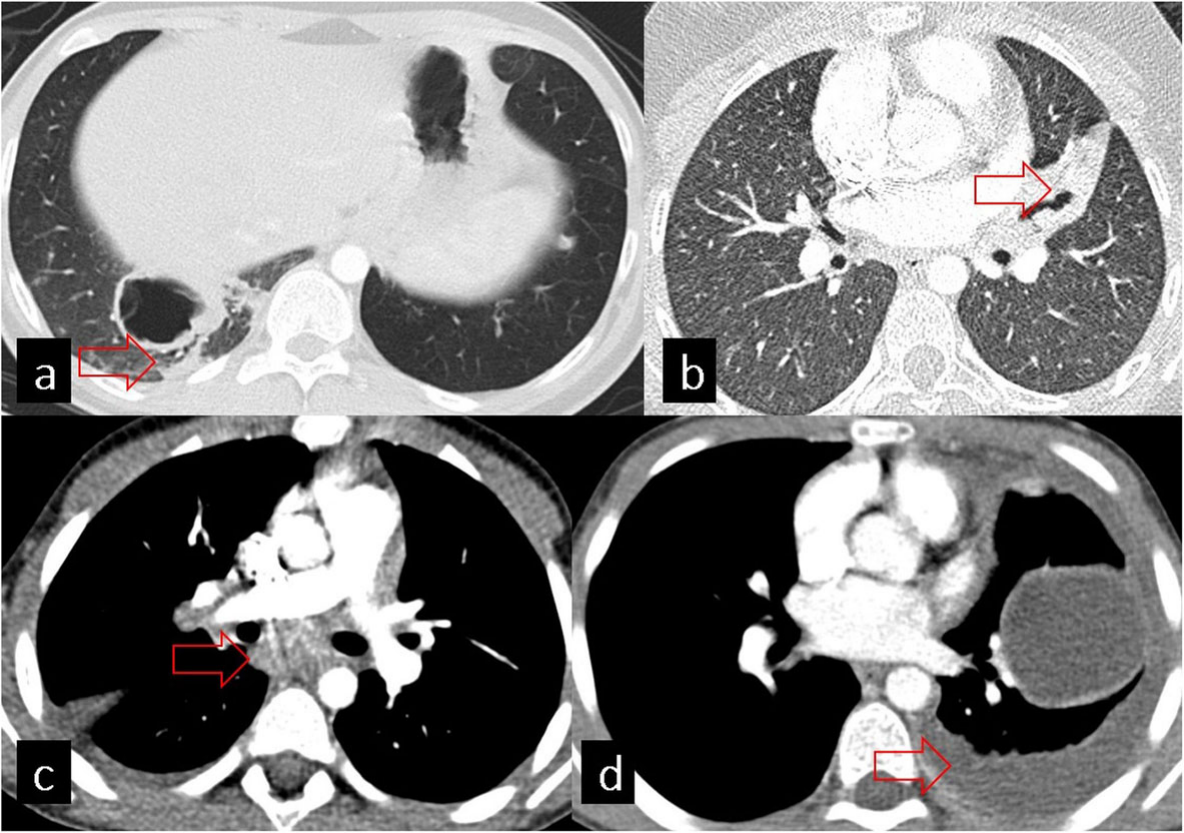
Axial CT images in the lung (a, b) and mediastinal (c, d) windows show associated findings of HC. a Atelectasis. b Bronchiectasis. c Mediastinal lymphadenopathy seen especially in hydatic cyst with superinfection. d Pleural effusion
